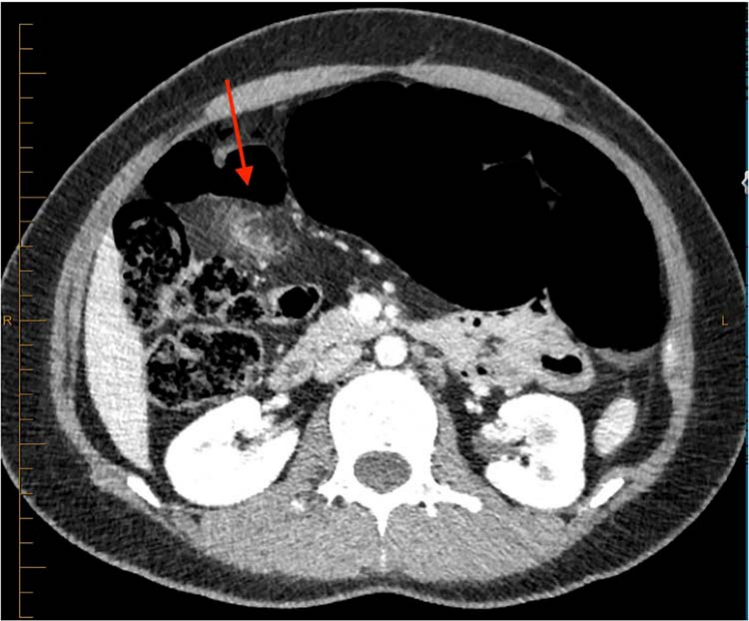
CT abdomen pelvis in 2019 (axial).
